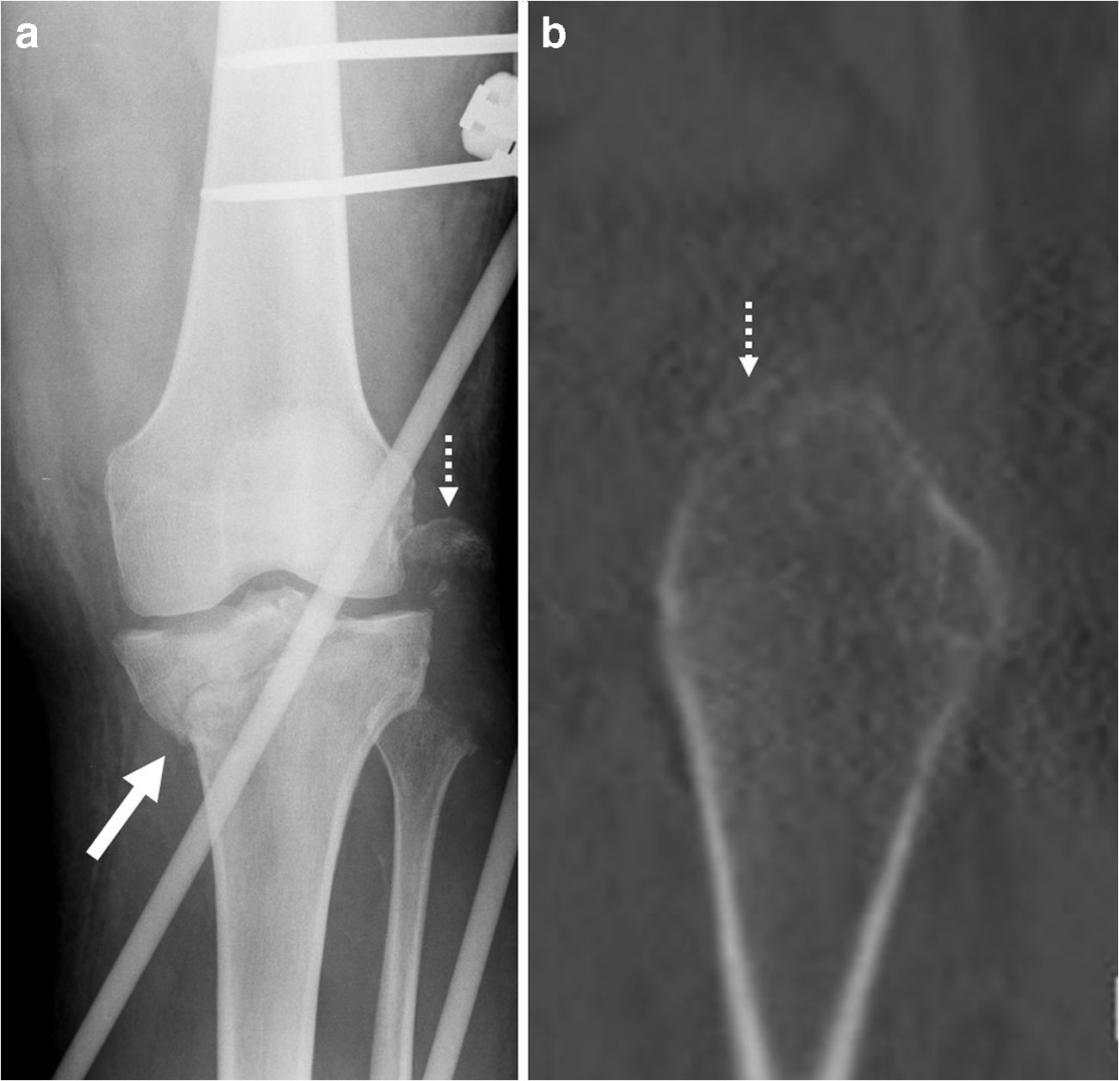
Fibular avulsion fragment sizes ranged in our study from large fragments clearly visible also on radiographs (a) to thin curved bone slivers barely seen on CT images (b). The radiograph (a) belongs to a 46-year-old male patient who fell on the stairs; and the sagittal CT image (b) is of a 17-year-old male patient who had a traffic accident while driving a moto-scooter. Both patients had fracture type of B3.3
